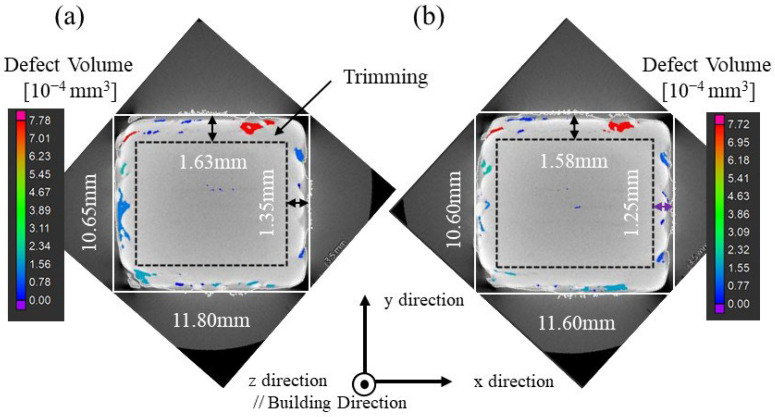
X-ray images of (a) uninoculated and (b) inoculated SUS316L samples manufactured at a laser power of 400 W.
